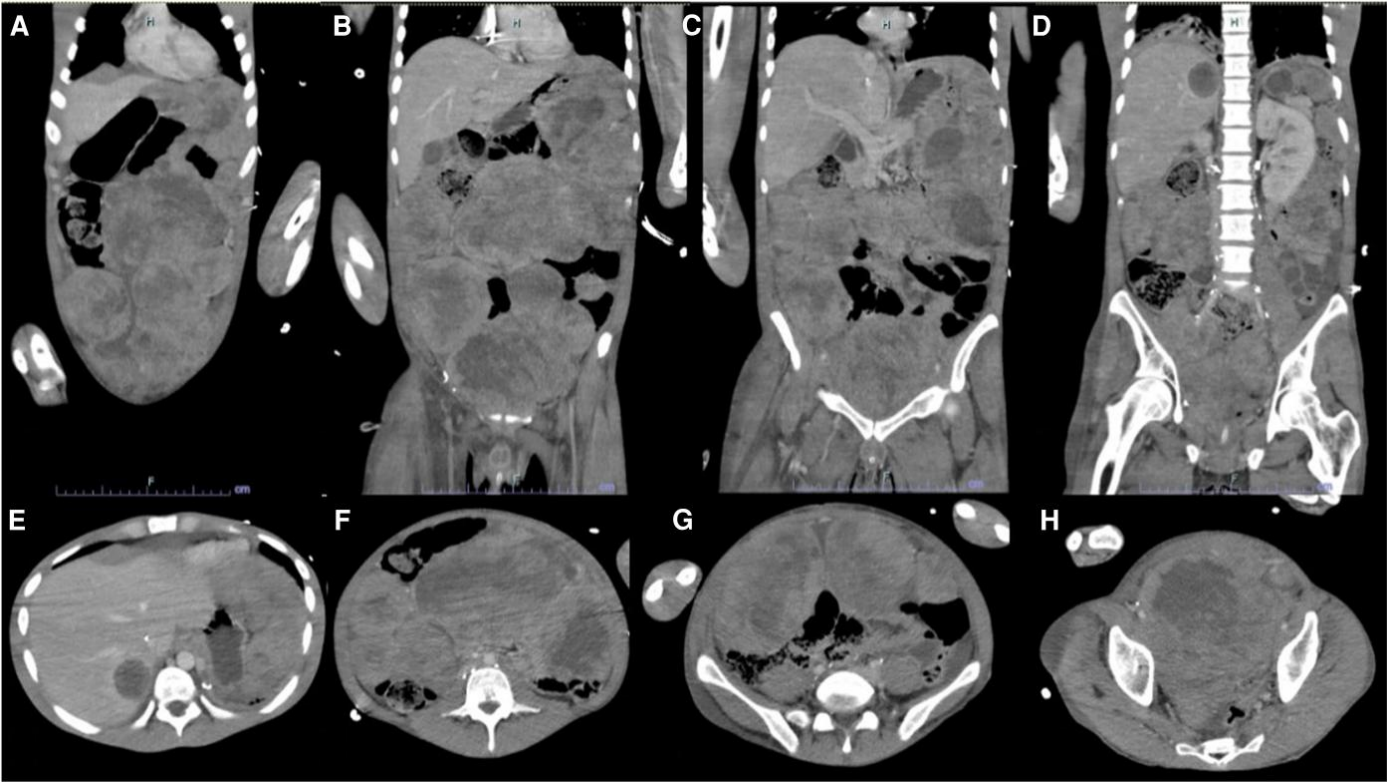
(A-H) Abdomen and pelvis computed tomography from presentation notable for significant abdominopelvic and hepatic lesions consistent with known metastatic embryonal rhabdomyosarcoma. There is also moderate right renal hydronephrosis due to mass effect that did not require decompression.
